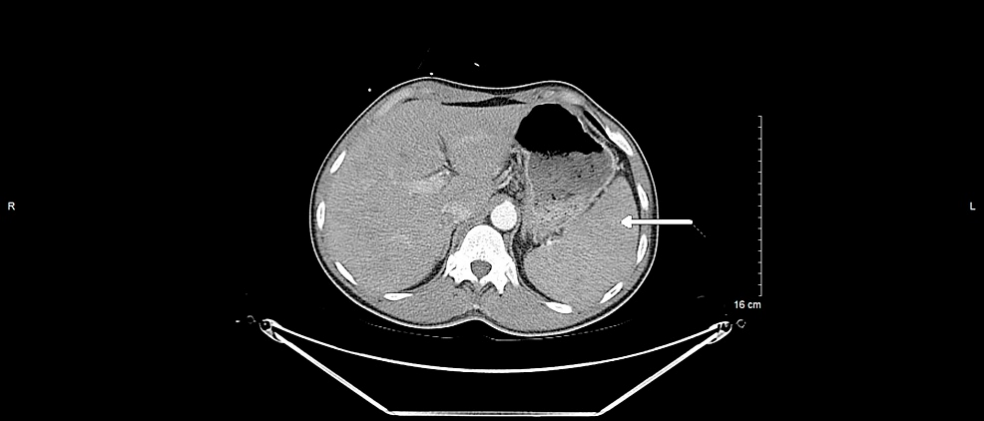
Computed tomography (CT) scan of the chest, abdomen, and pelvis depicting mild splenomegaly. White arrow shows the mild splenomegaly.
